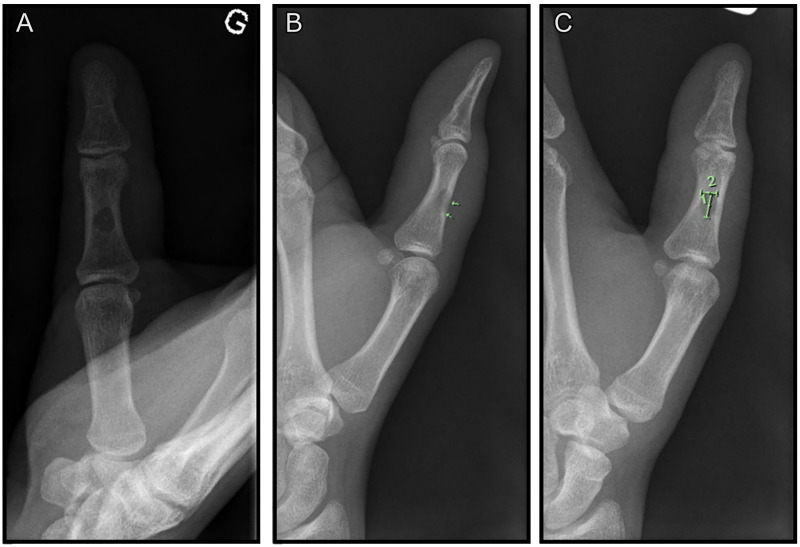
X-ray of the left thumb: antero-posterior view (A), lateral view (B), and oblique view (C); showing a lytic lesion of the mid proximal phalanx. Green annotations show its dimension of 0.68 cm [1] × 0.45 cm [2].
